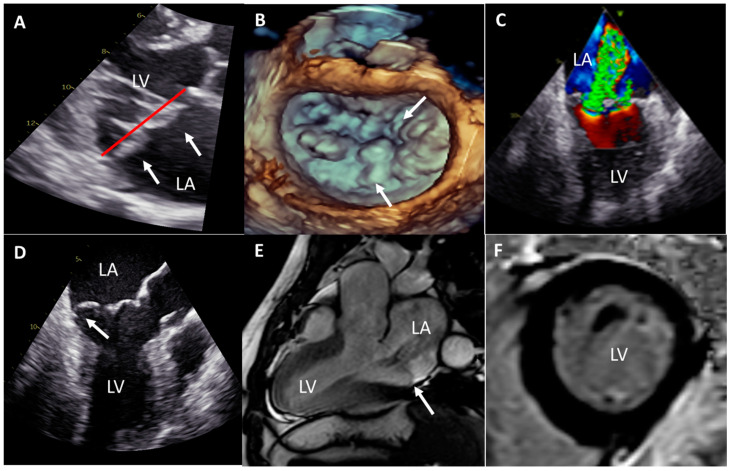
Imaging of the mitral valve prolapse and of the left ventricle. (A)Trans-thoracic echocardiography, parasternal long-axis view, demonstrating thickened bi-leaflet prolapse (white arrows) of the mitral valve. The red line identifies the mitral annular level. LA: left atrium; LV: left ventricle. (B) Trans-esophageal 3D echocardiography of the mitral valve, showing the detailed anatomy of the multi-scallop, bi-leaflet prolapse (white arrows) of the Barlow′s-like mitral valve. (C) Trans-esophageal Color Doppler echocardiography (bi-commissural view), showing the severe (multi-jet) mitral regurgitation. (D) Trans-esophageal echocardiography (three-chamber view) showing the prolapse of the mitral valve posterior leaflet and mitral annular disjunction (MAD) (white arrow). (E) CMR (three-chamber view cine) showing the prolapse of the mitral valve with mild MAD and dilated LV. CMR: cardiac magnetic resonance. (F) CMR (delayed enhancement scan of one representative slice of the short axis at the level of the papillary muscles), showing no evidence of macroscopic myocardial fibrosis/scar (black myocardium without any white signal).
